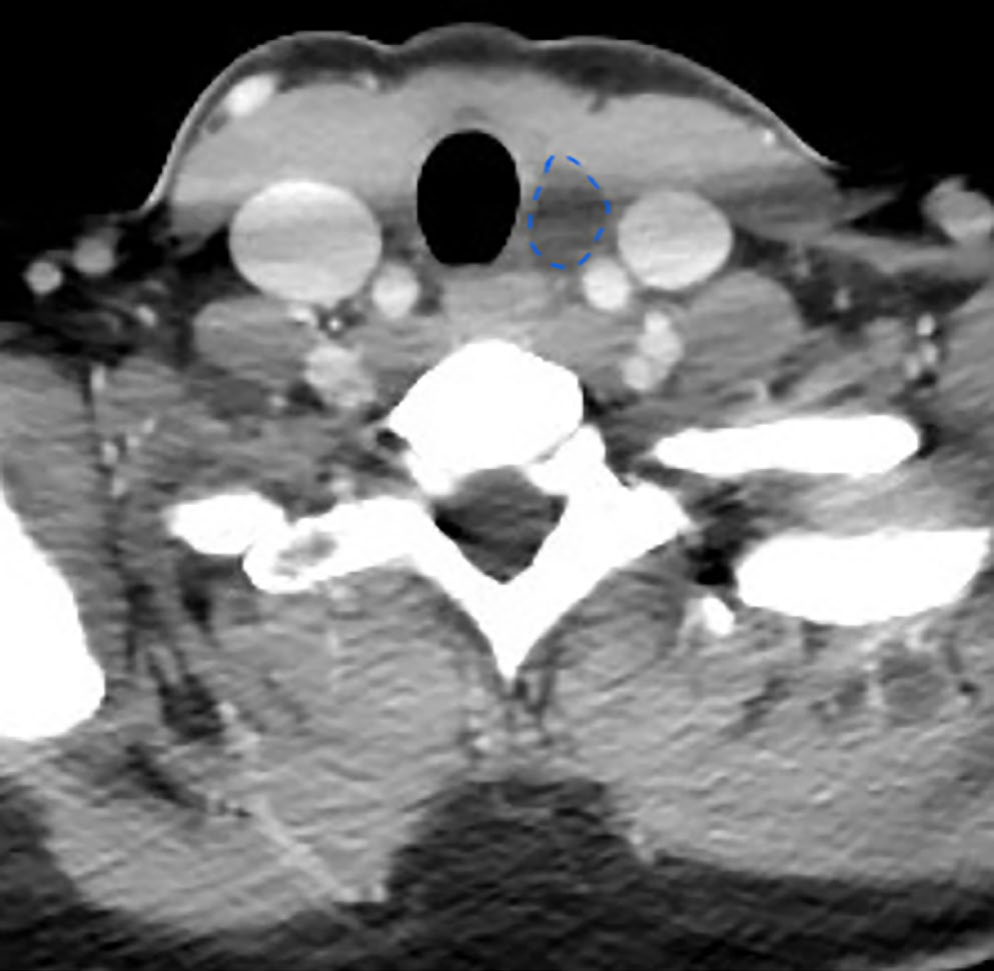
CT axial scan of the mass in the left post-thyroidectomy cavity. The dotted blue line surrounds the mass
